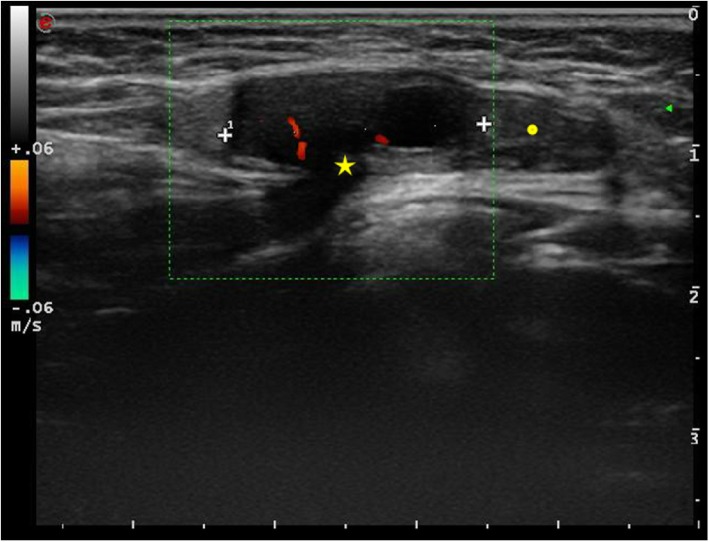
The ultrasound imaging shows a 3 cm hernial sac bulging through a 5 mm breach (star) from the inguinal canal. The hernial pouch consisted of adipose tissue in the cranial portion (point), followed by a solid elliptical formation that appears vascularized at color doppler
